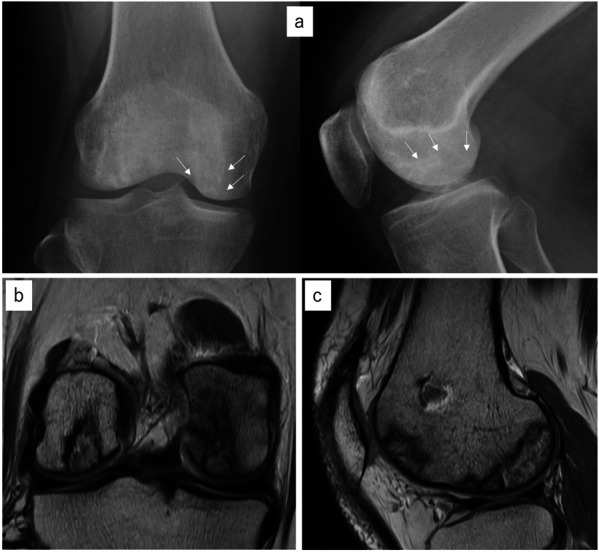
Preoperative imaging findings of the case (Patient No. 7, right knee). Fig. 2-A Anteroposterior and lateral plain radiographs demonstrating focal sclerotic changes in the medial femoral condyle (white arrows). Fig. 2-B Coronal proton density-weighted MRI showing osteonecrotic lesions involving the weight-bearing regions of both femoral condyles. Fig. 2-C Sagittal proton density-weighted MRI of the medial femoral condyle demonstrating extensive osteonecrosis in the posterior aspect of the condyle. MRI = magnetic resonance imaging.
